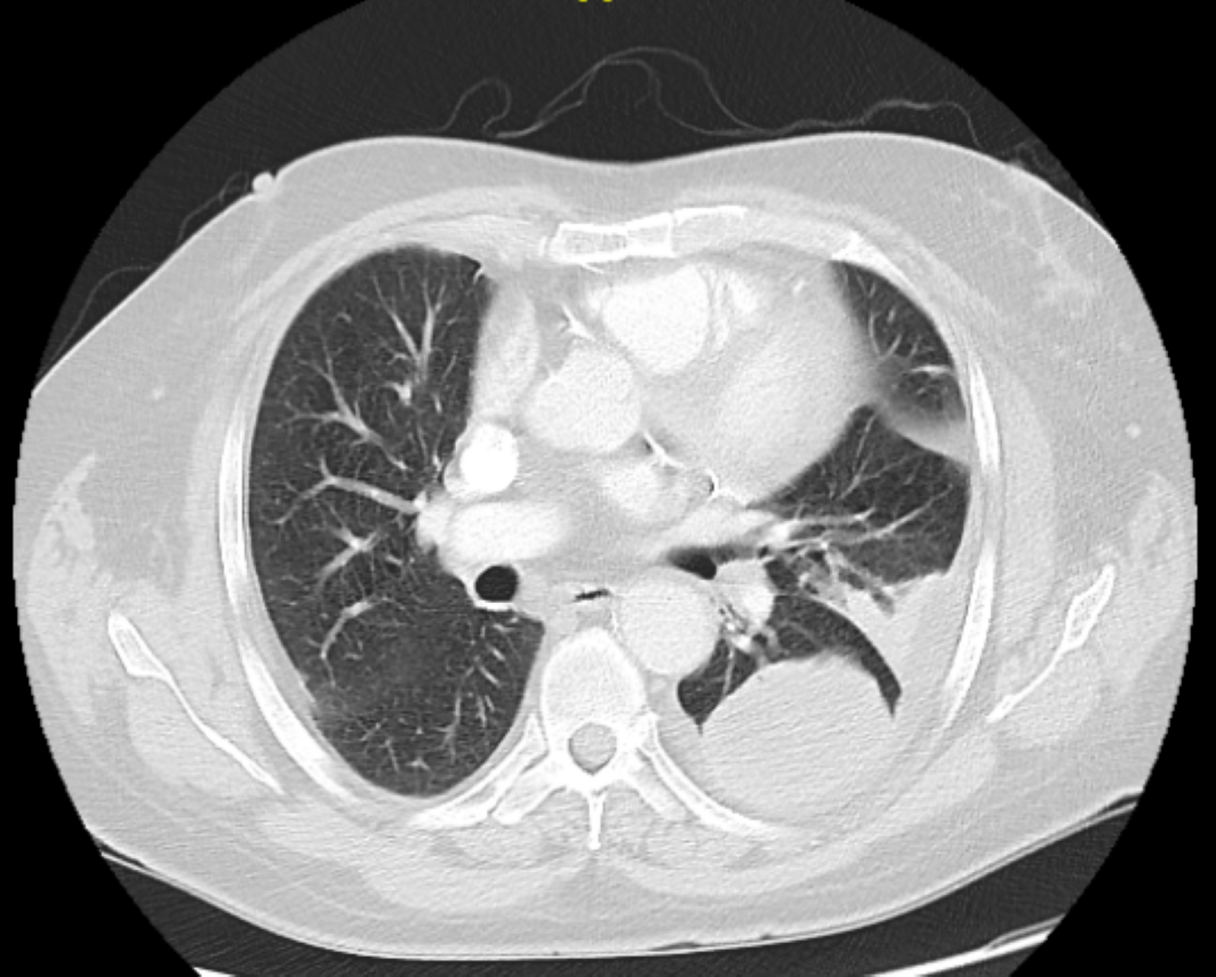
CT scan of the chest with and without contrast, shows a large round mass-like lesion measuring 6 cm in the left lower lobe of the lung without evidence of nodal involvement or metastatic disease.
